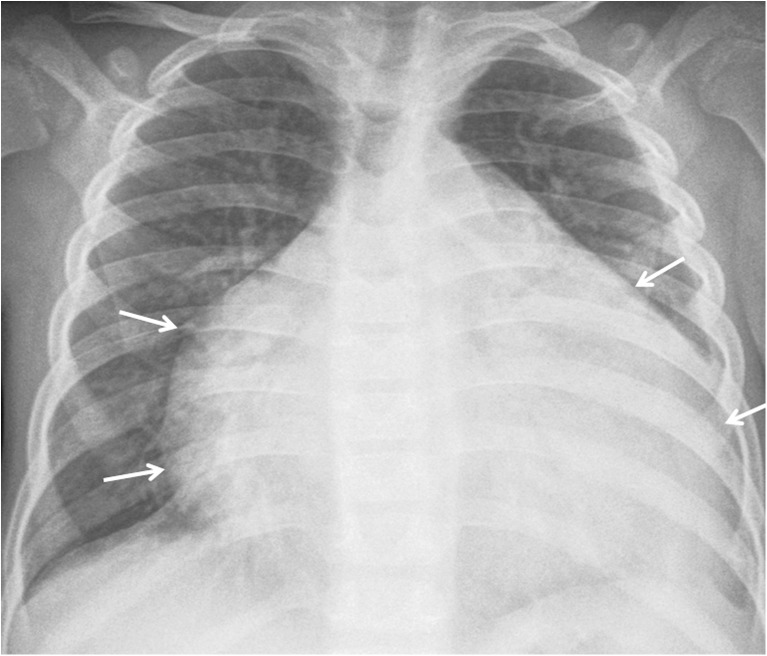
Tuberculous pericarditis in a 6-year-old boy. Anteroposterior radiograph of the chest shows globular enlargement (*arrows*) of the cardiac silhouette (water bottle sign)
